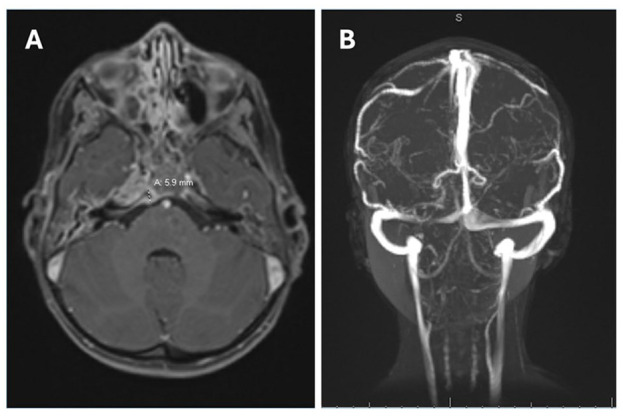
MRV head on admission. Paranasal sinus disease without invasive changes. There are bilateral middle ear and mastoid effusions with extension to the petrous apex with septal enhancement. At the rigth petrous apex and clivus, there is enhancement, patchy edema, loss of normal fine septations, and smooth osseous margins. A 5.9 mm extra-axial collection was appreciated on the right side of the prepontine cistern overlying the petroclival synchondrosis, which with associated diffusion restriction, was in keeping with a small abscess. There was asymmetric increased T2 FLAIR and postcontrast enhancement involving the wall of the petrosal and proximal cavernous segments of the right internal carotid arter with moderate luminal narrowing within these segments. No cerebral venonus sinus thrombosis was appreciated.
